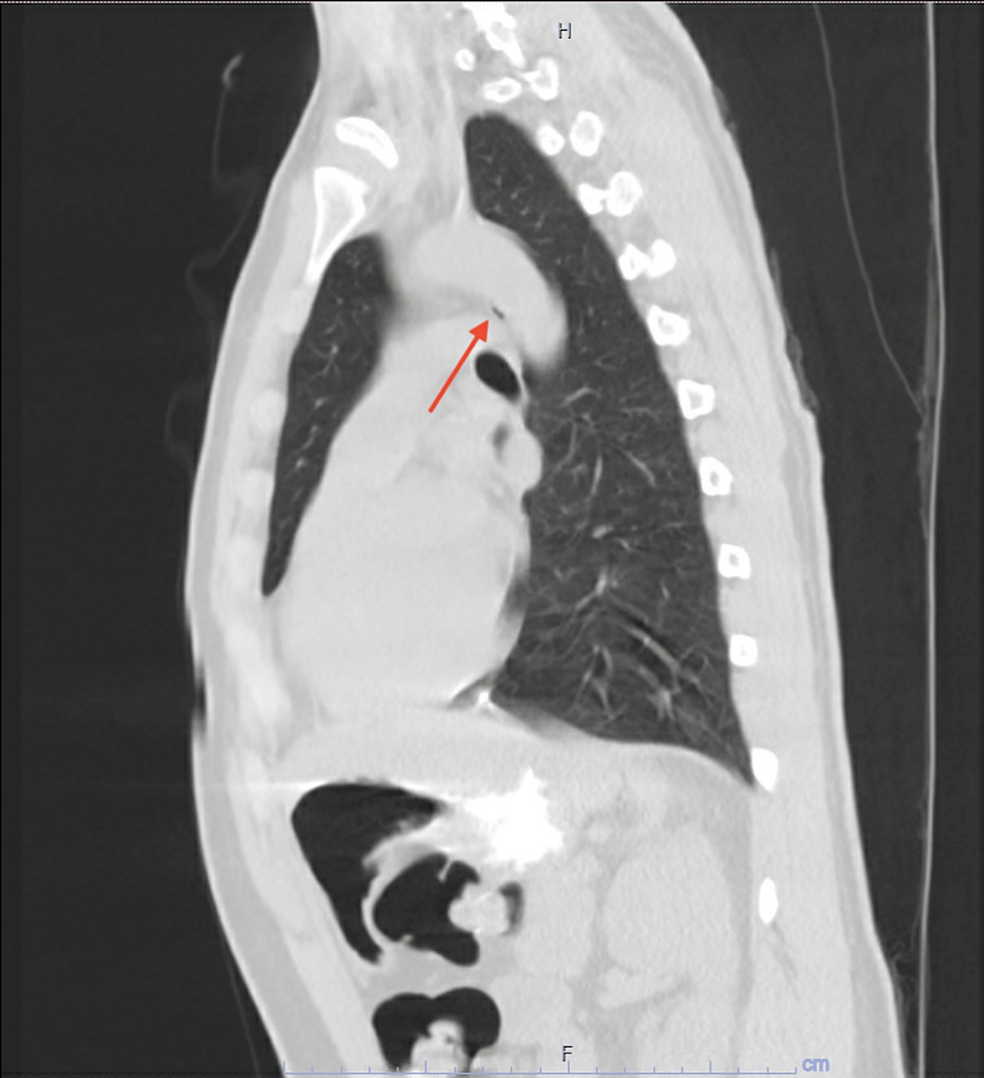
Sagittal view of CT abdomen Collections of air surrounding the esophagus (red arrows)
